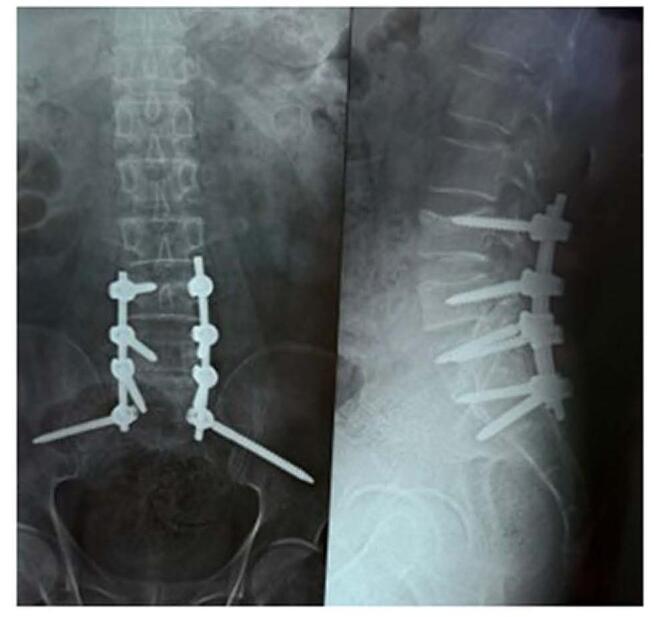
X-ray spine antero-posterior and lateral views at follow up showing implants in situ.
